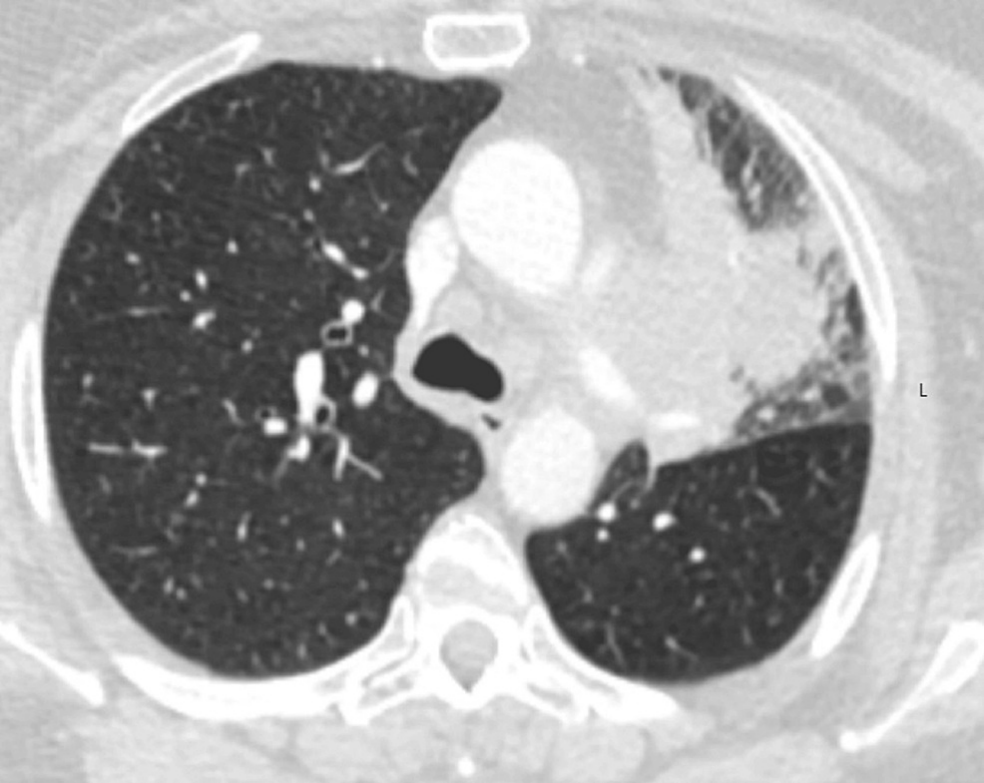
Chest computed tomography scan showing the mass on the left side
